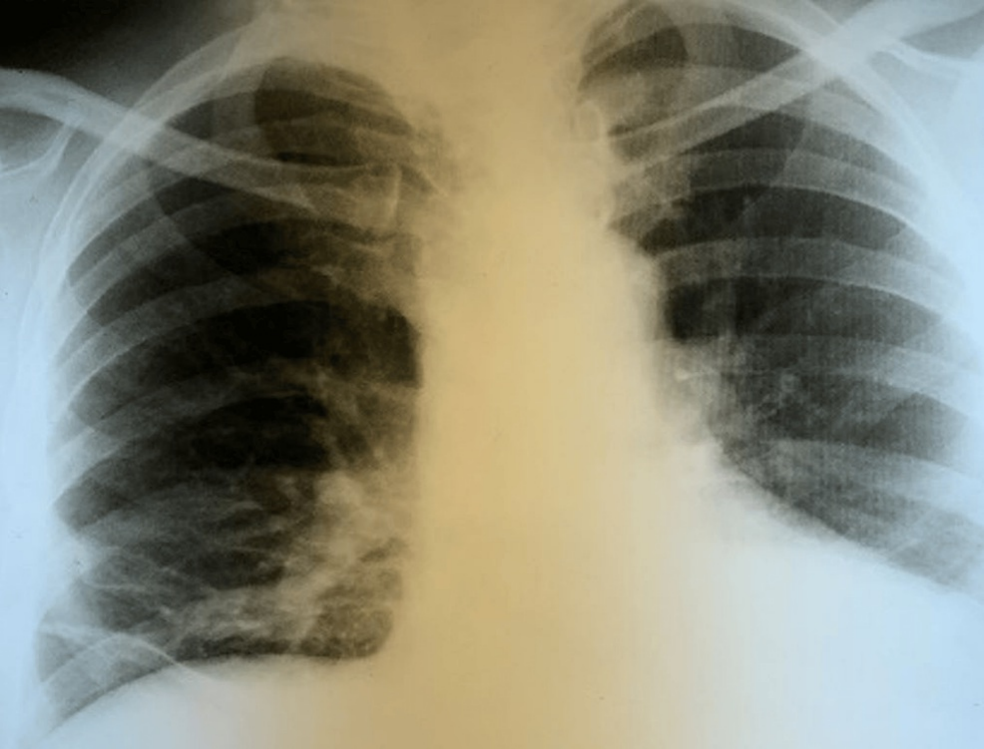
Chest X-ray on 14 August 2019
